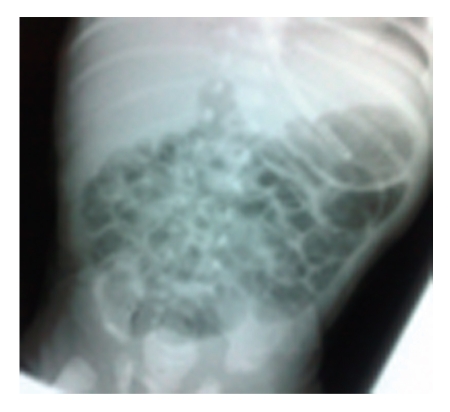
Abdominal radiograph that shows small intestine dilated loops in central position and a gasless distal part of intestine.
